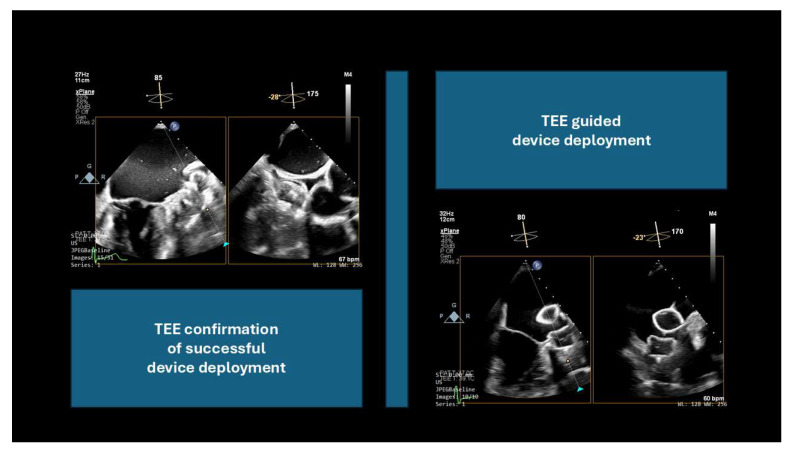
Left atrial appendage transesophageal echocardiography imaging: device deployment and positioning confirmation.
